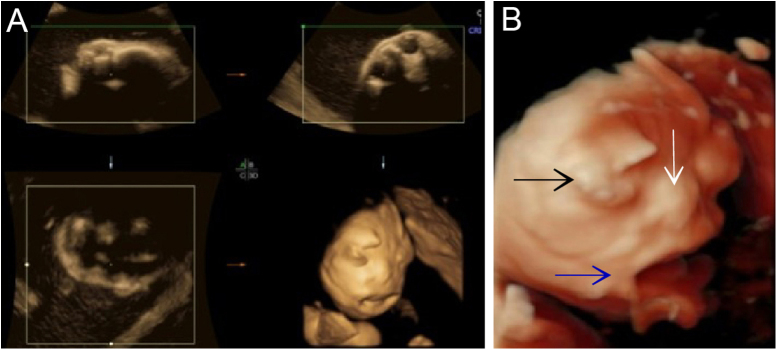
(A) 3-D ultrasound: the image of the face of the fetus. (B) 3-D ultrasound: eyelid edema and ectropion (black arrow); Hypoplastic nose (white arrow); Eclabium and anterior tongue protraction (blue arrow)
